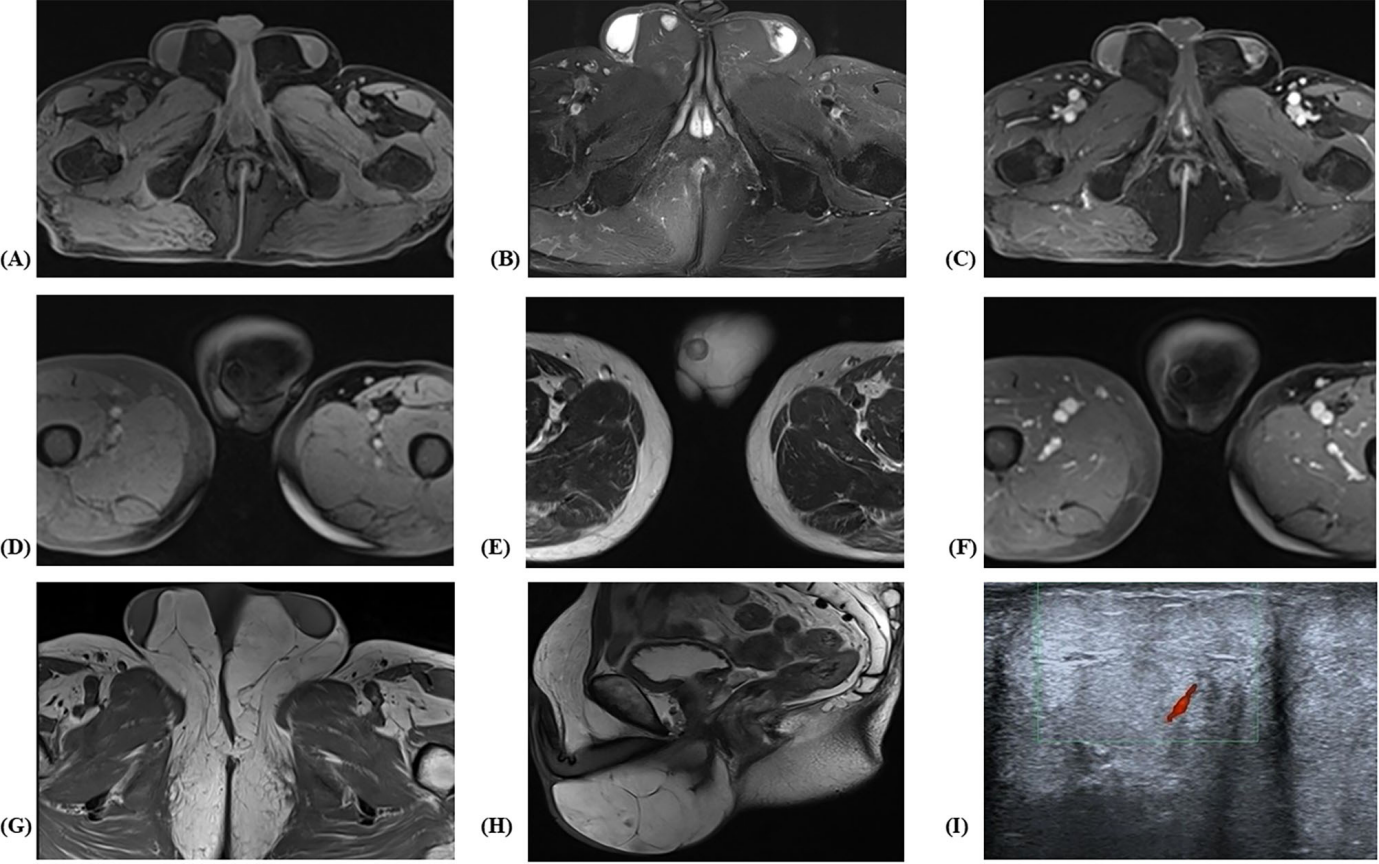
Axial T1WI-FS (A), T2WI-FS (B) and T1WI-enhanced (C) MR images of the scrotum showed the nodule in right scrotal sac was hyperintense on T2WI and hypointense on T1WI. The small nodule exhibited mild contrast enhancement. Axial T1WI-FS (D), T2WI-FS (E) and T1WI-enhanced MR (F) images showed the nodule in left scrotal sac was fat signal with ring-like low signal intensity and mild peripheral (ring-like) enhancement. Axial T1WI-FS (G) and sagittal T2WI (H) demonstrated fat deposition of the perineum and the root of the penis with marked septations. Scrotal ultrasound (I) showed two morphologically and dimensionally normal testicles encircled by hyperechoic masses which involved perineum.
